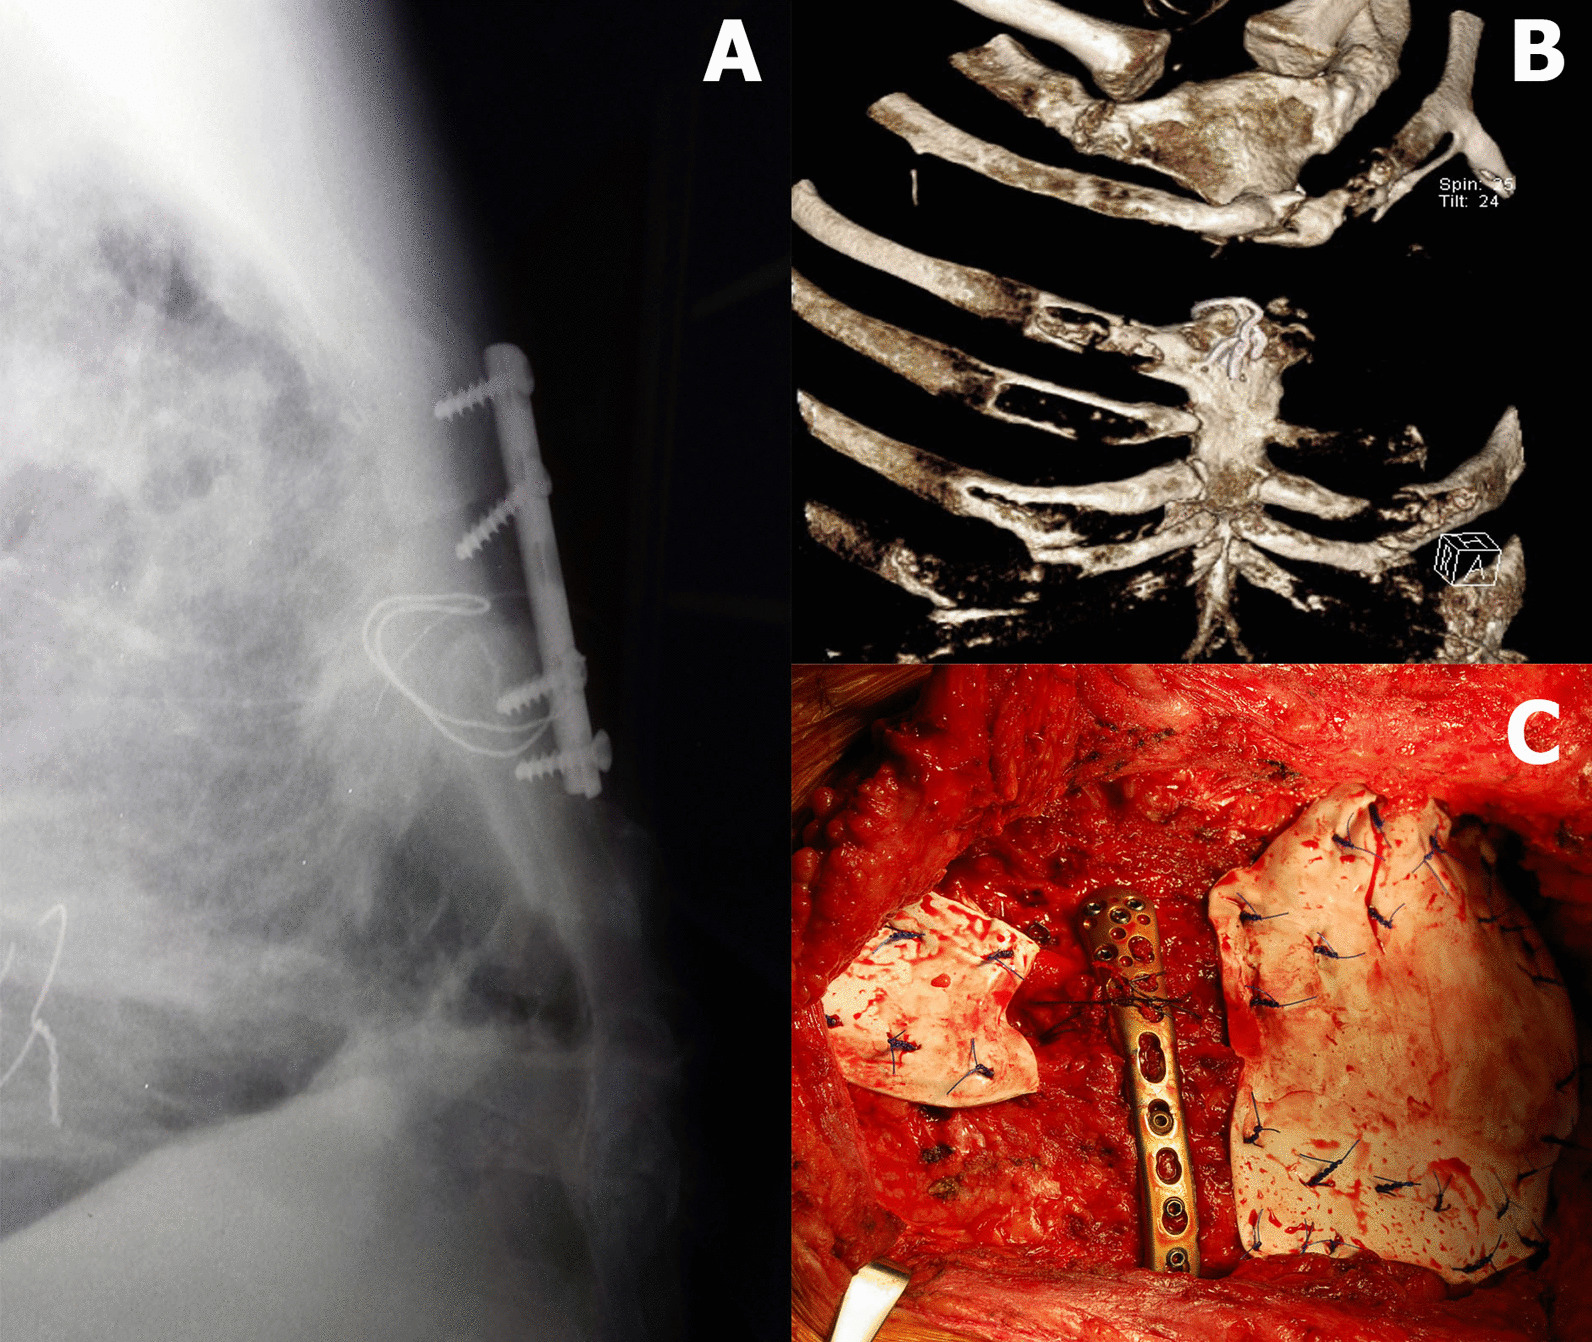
a A failed fixation of the transverse sternal nonunion with one-third tubular plate and tension wiring. b 3D reconstruction of the CT showing a big chest wall defect resulting from sternal nonunion and rib resection. c Intraoperative view of the sternal fixation with a locking tibial plate and closure of the remaining chest wall defects with two Gore-Tex dual mesh membranes
